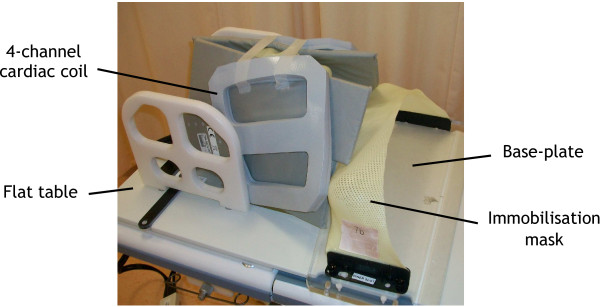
MRI acquired in the radiotherapy position with a 4-channel flexible surface coil positioned laterally.
